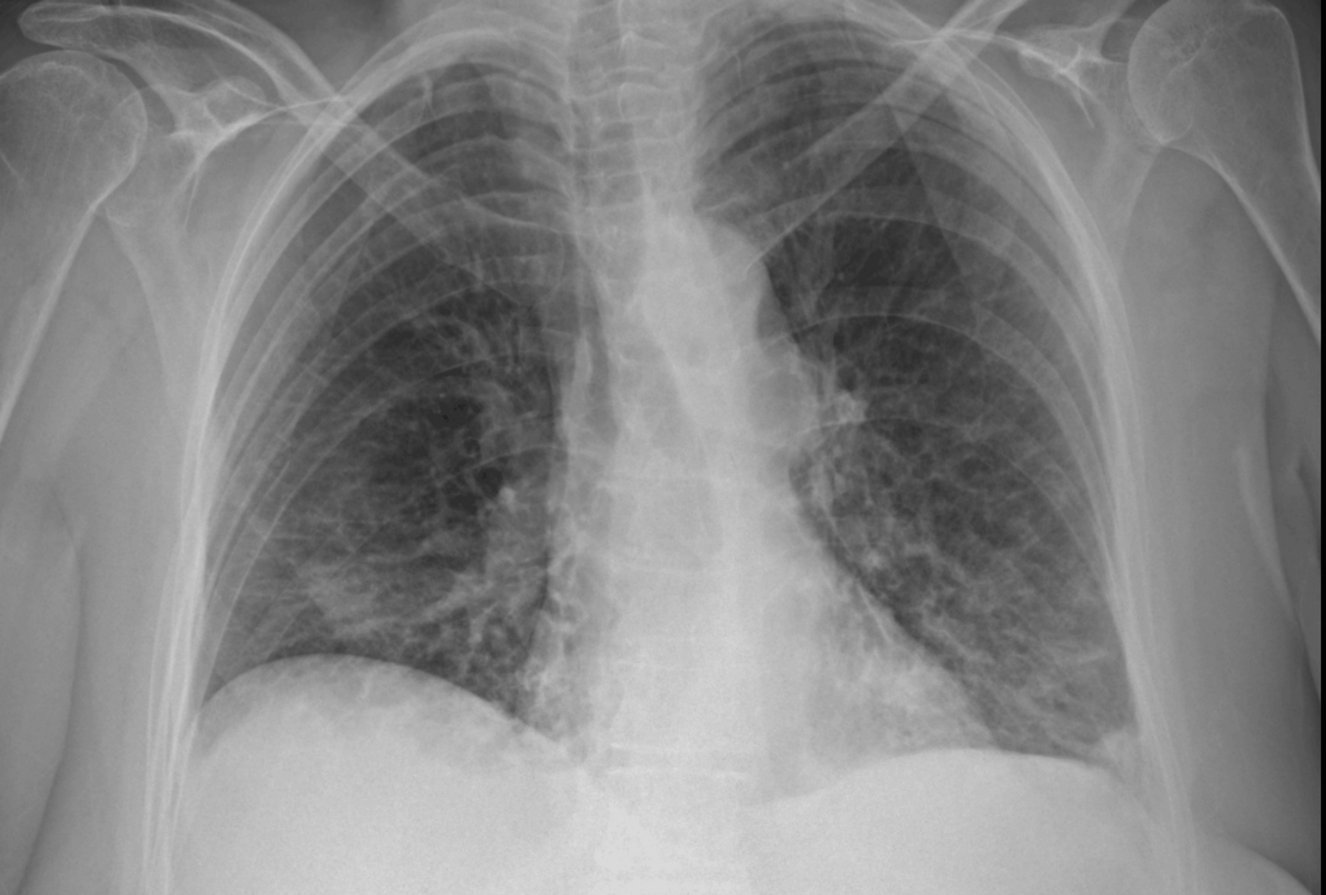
Chest radiograph on day 36 showing bilateral ground-glass changes in the lower hemithorax.
